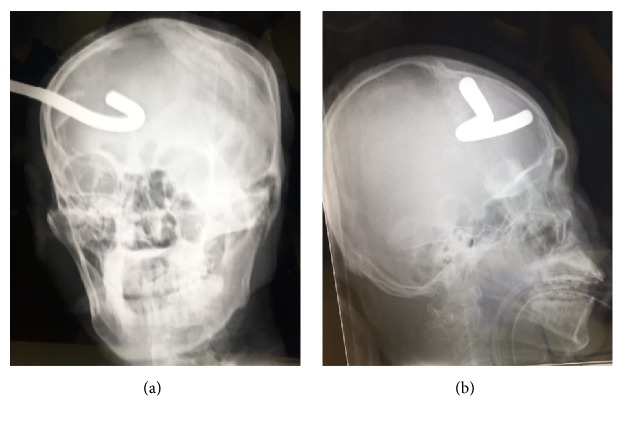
(a) Plain X-ray of the skull (A-P view) showing a hook-shaped metal bar on the right side. (b) Plain X-ray of the skull (R-L view) showing the metal bar in the frontal region.
